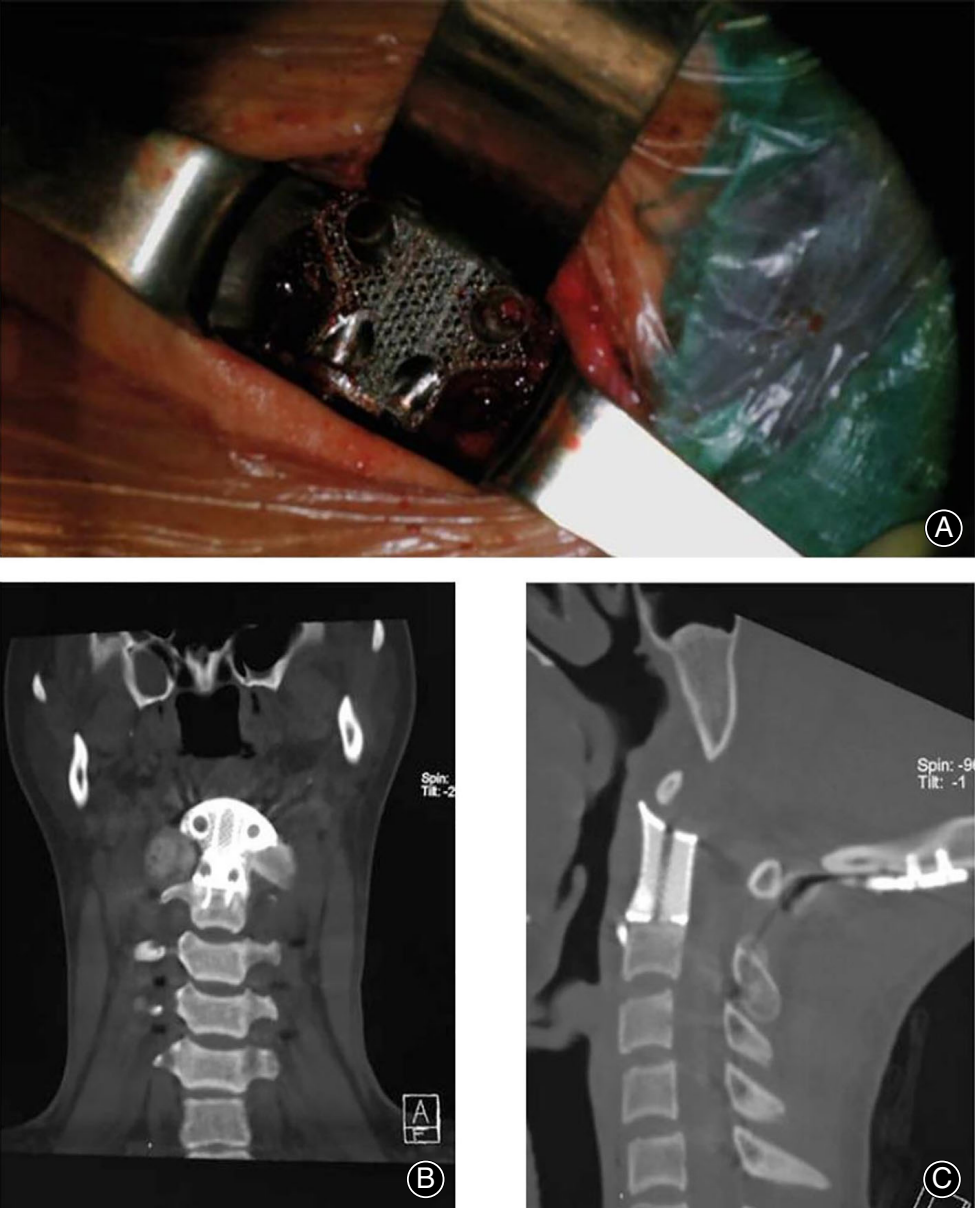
(A) Intraoperative view of the 3D‐printed artificial vertebral body in place during the anterior procedure. (B) Coronal CT reconstruction of the 3D‐printed artificial vertebral body at the 3‐month follow‐up visit. (C) Sagittal CT reconstruction of the 3D‐printed artificial vertebral body at the 3‐month follow‐up visit.^72^ (Taken from Cai *et al*.^72^)
